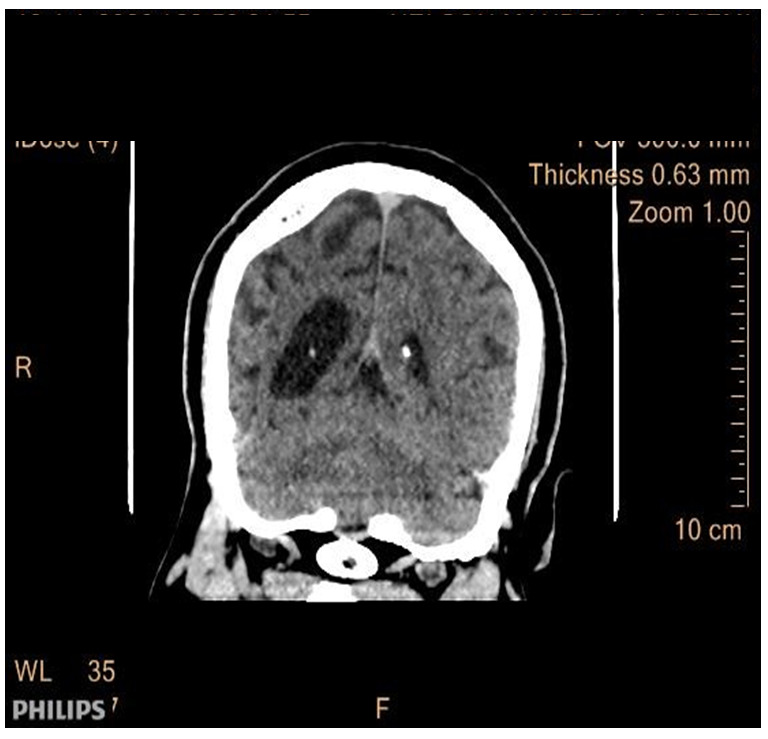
CT scan of the brain (coronal view). Shows a notable atrophy of the right cerebral hemisphere with enlargement of the ipsilateral lateral ventricle.
